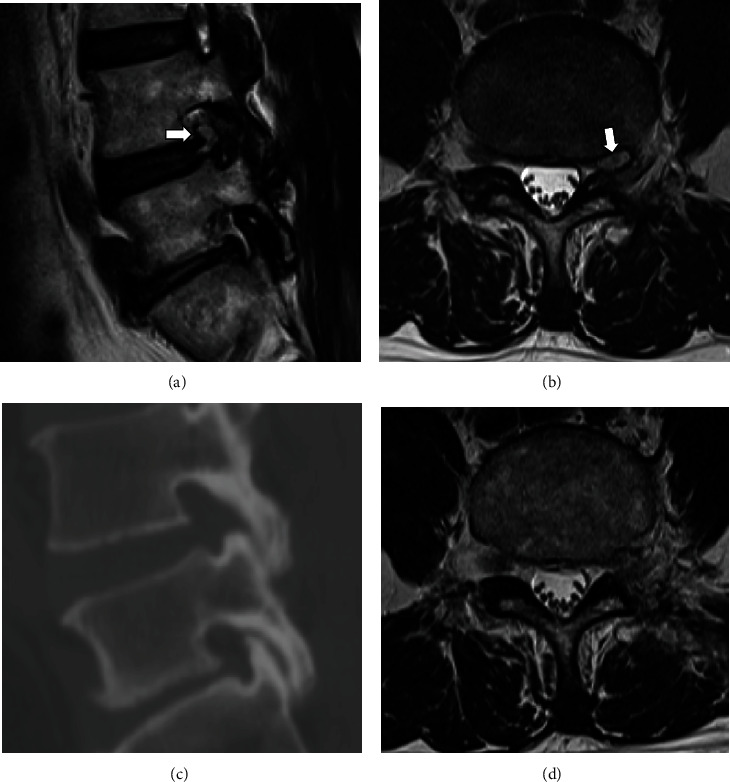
Preoperative (a) sagittal, (b) axial T2-weighted MRI of disc herniation (white arrow) that recurred in the same area 3 months after decompression through a far lateral approach. (c) Preoperative CT scan determines the extent of bone resection performed in the previous surgery. (d) Postoperative MRI confirm that the herniated disc has been removed.
